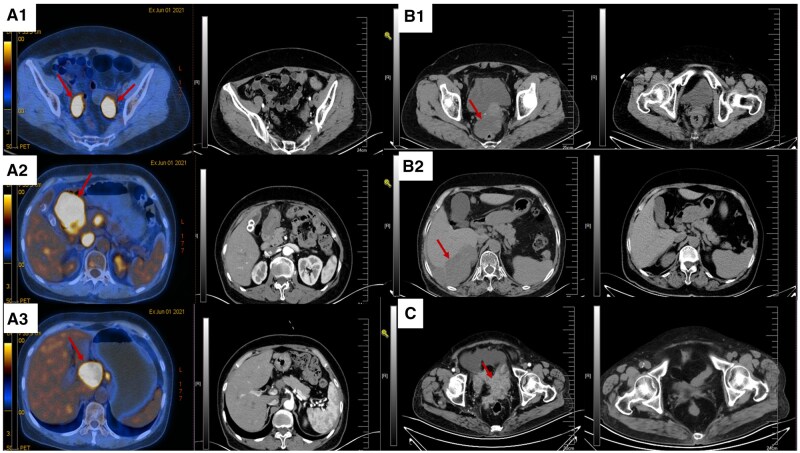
Comparison of CT images before and after treatment. (A) 1-3. Patient P6. (B) 1-2. Patient P9. (C) Patient P10. The lesion indicated by the arrow corresponds to the baseline lesion prior to treatment.
